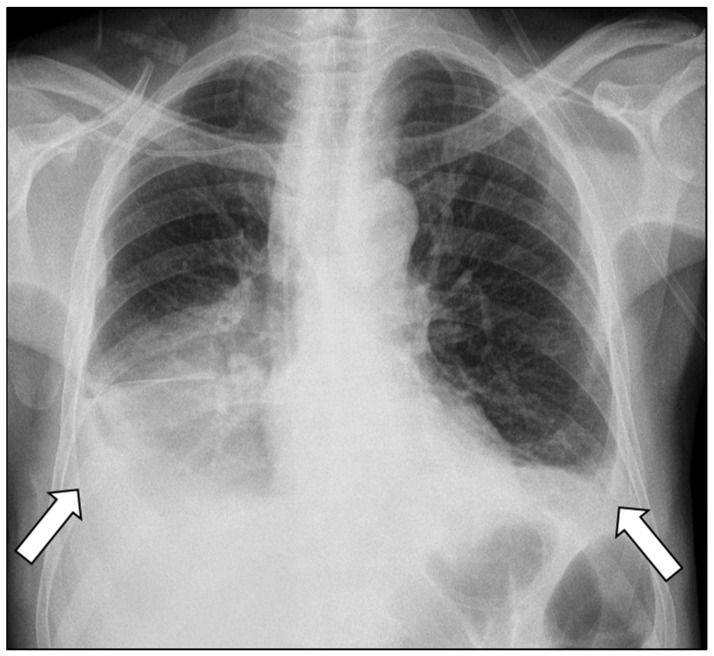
Chest radiograph demonstrating bilateral pleural effusions (arrows), more pronounced on the right side.
